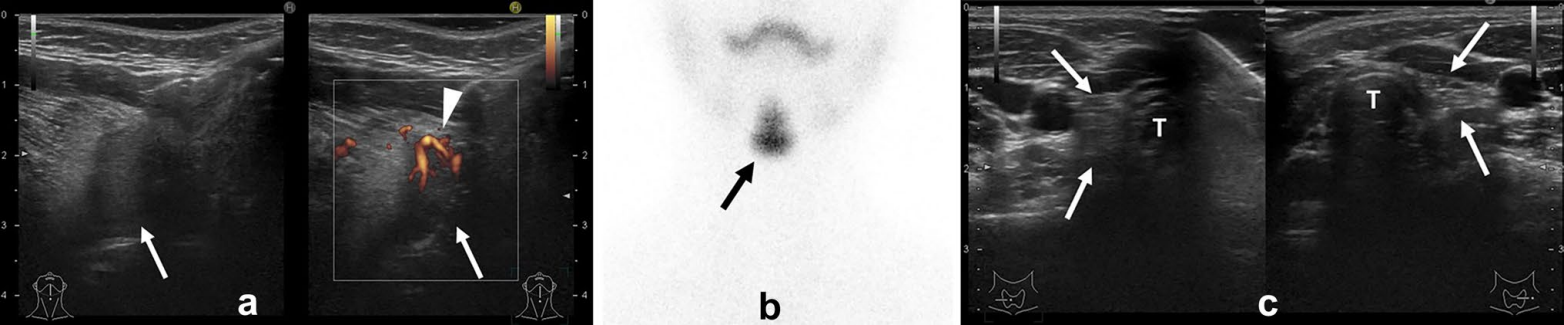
Five-year-old girl with ectopic thyroid gland. a Ultrasound showed a nodule with lower echogenicity than the surrounding tissue at the base of the tongue. Power Doppler showed blood flow in the nodule. b 99mTc-O4- Scintigraphy revealed uptake of lingual thyroid. There was no uptake of normal thyroid where should be. c Ultrasound showed slight hyper-echoic areas (arrows) where the thyroid gland should be. These slight hyper-echoic areas were considered as fat or interstitium in the dead space. Normal thyroid tissue was not able to be detected around there. T: trachea
